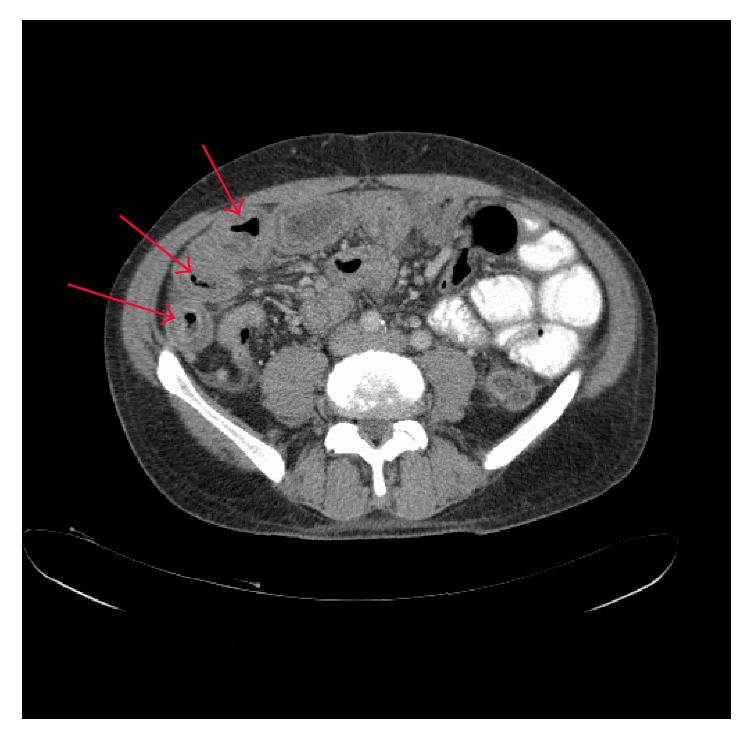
CT abdomen axial view showing thickening of the small bowel wall as indicated by the arrows. March 2011.
